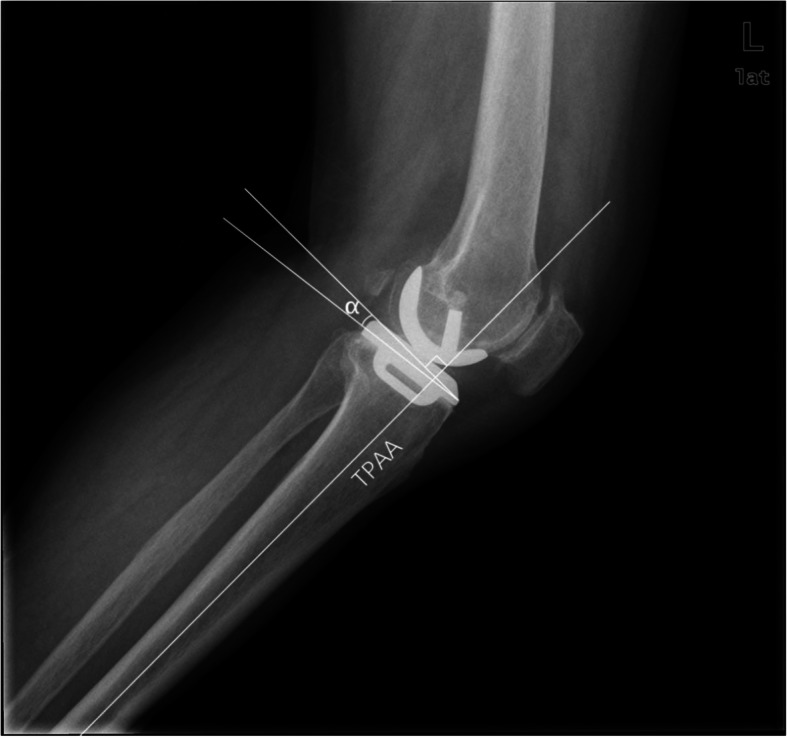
The measurement method on standardized knee radiographs in the lateral view. TPAA, the tibial proximal anatomical axis. Tibial posterior slope (PTS) was defined by the angle between the posterior inclination line of the medial tibial plateau and a line perpendicular to the TPAA, which is defined by α-angle
